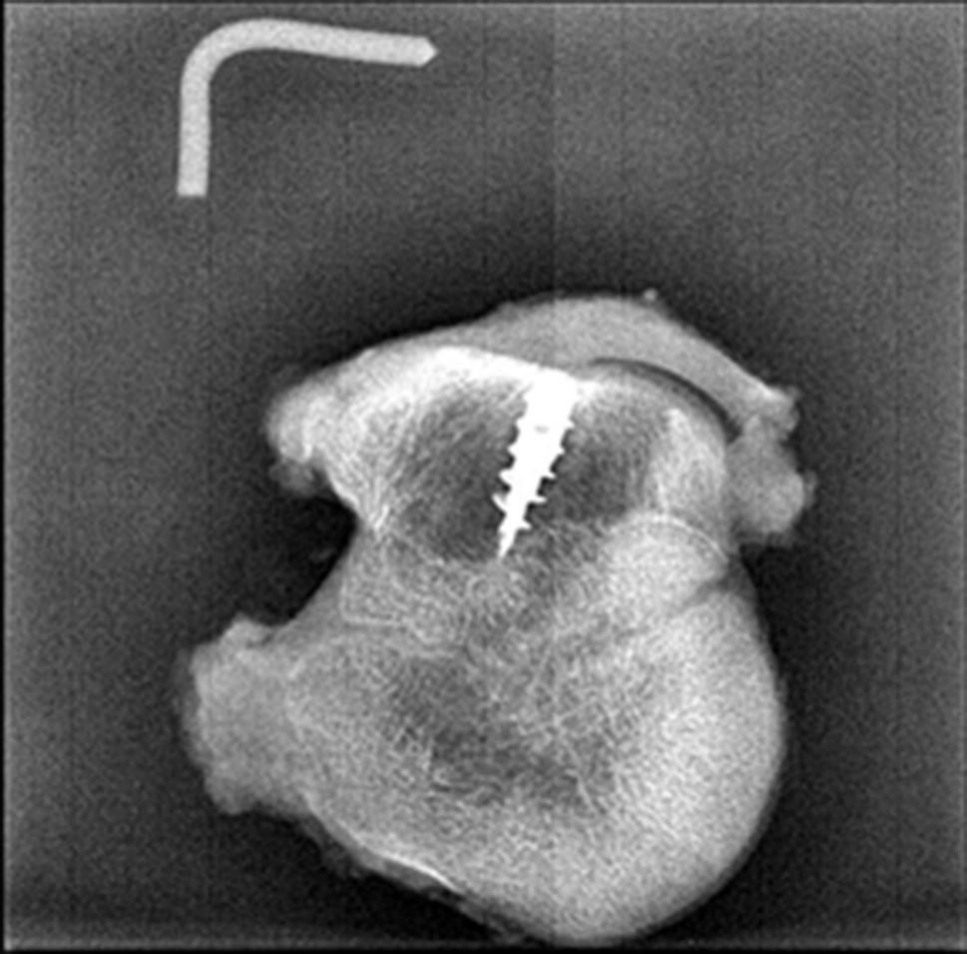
Postoperative X-ray revealing adequate positioning of the suture anchor and no displacement
